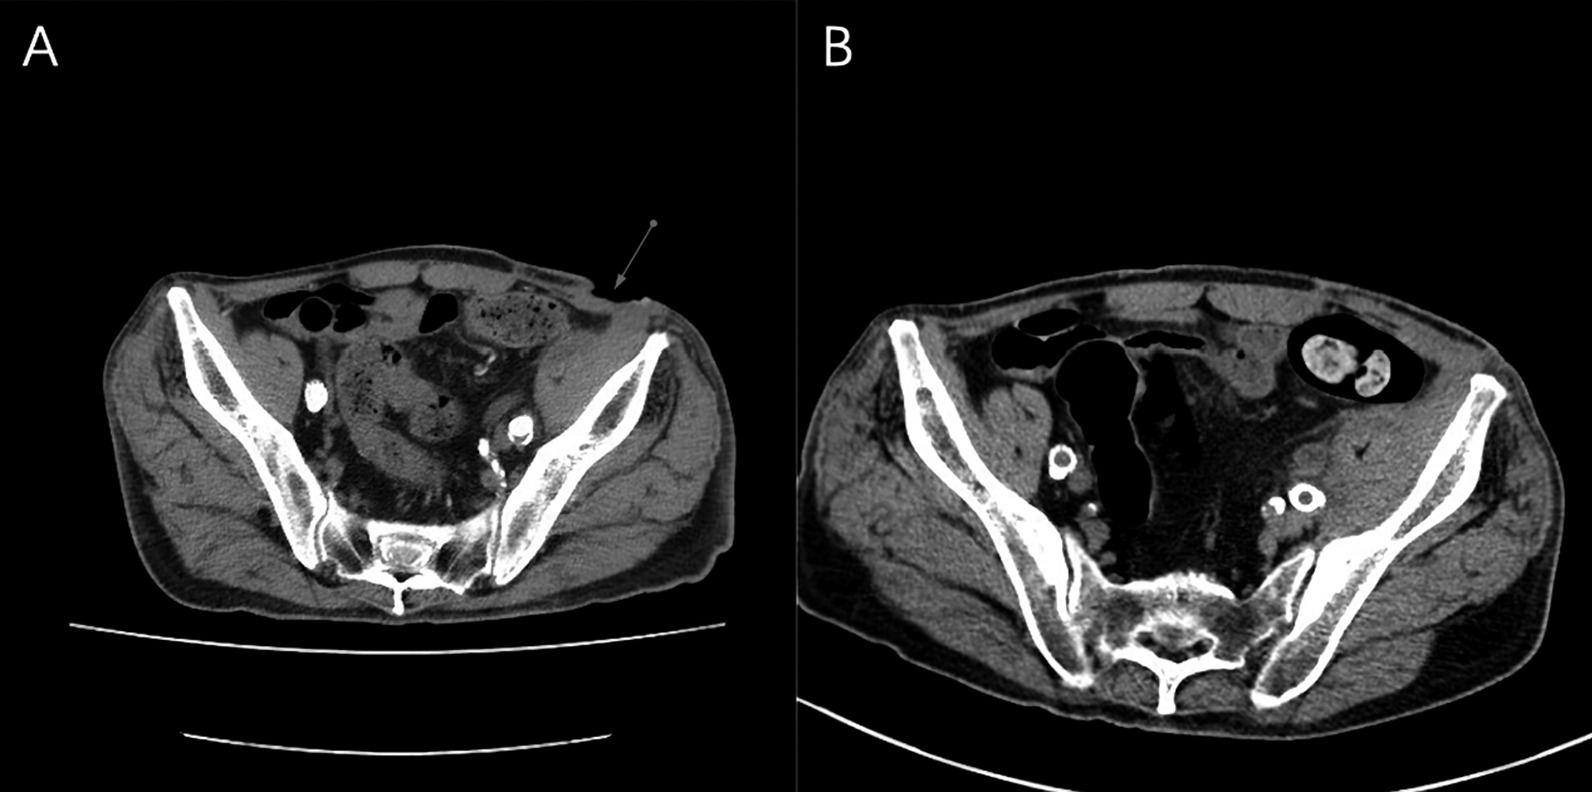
A and B were the abdominal CT before and after treatment, respectively. In figure A, the red arrow pointed to the obvious skin discontinuity, and the same part in figure B had healed
